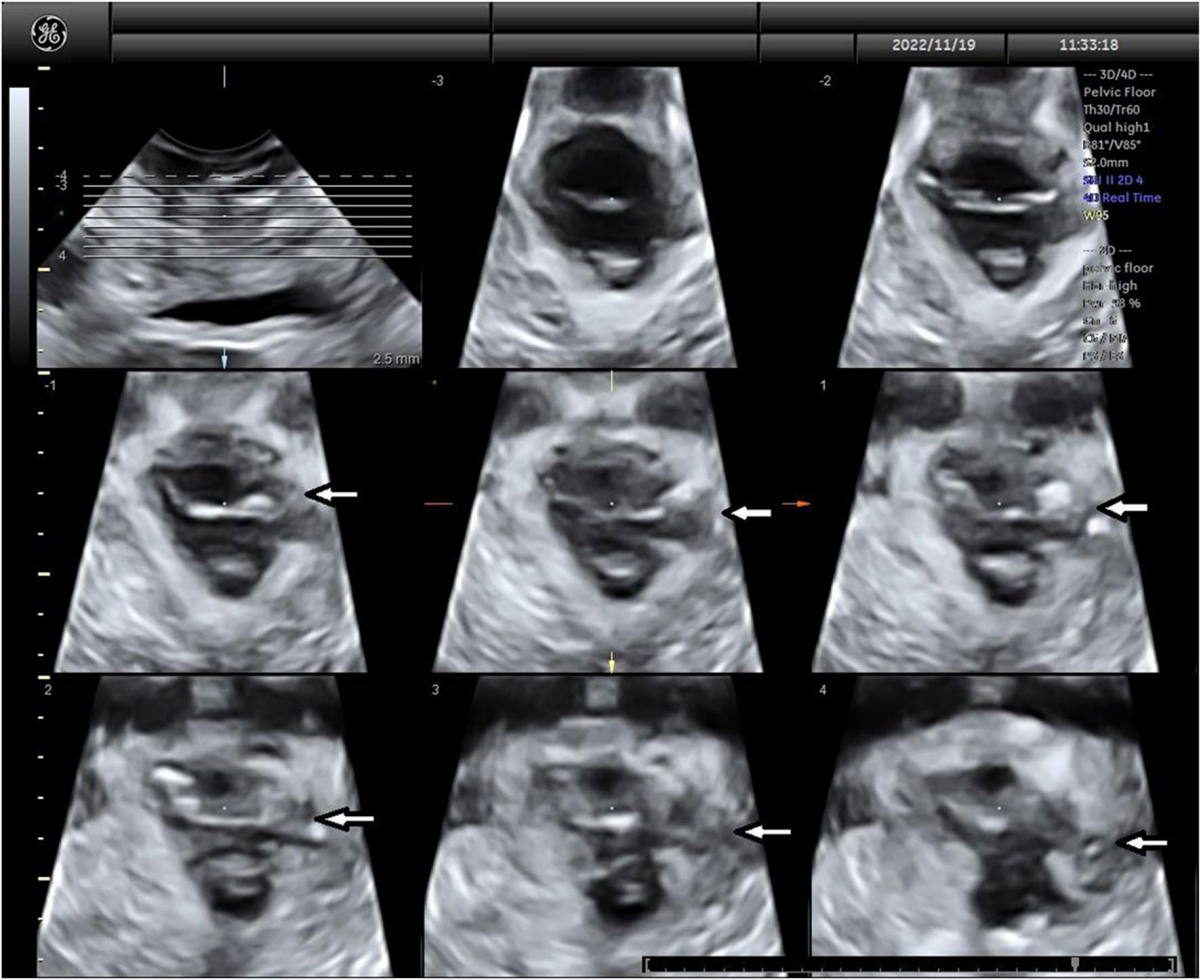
Transperineal tomographic ultrasound imaging of the levator ani muscle (*LAM*). Sequential-level ultrasound imaging reveals full left-sided avulsion (*white* *arrows*) of the levator ani pelvic floor muscle group 3 months after vaginal delivery. The avulsion injury appears as a frank interruption in the smooth arcing topography of the intact LAM complex seen on the contralateral (*right*) side in these ultrasound images
